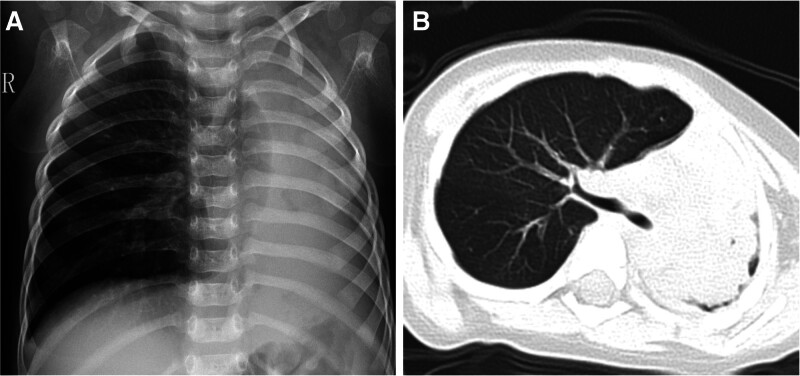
(A) Chest radiograph depicting atelectasis of the left lung; (B) chest computed tomography revealing a high-density image of the left main bronchi and atelectasis of the left lung.
